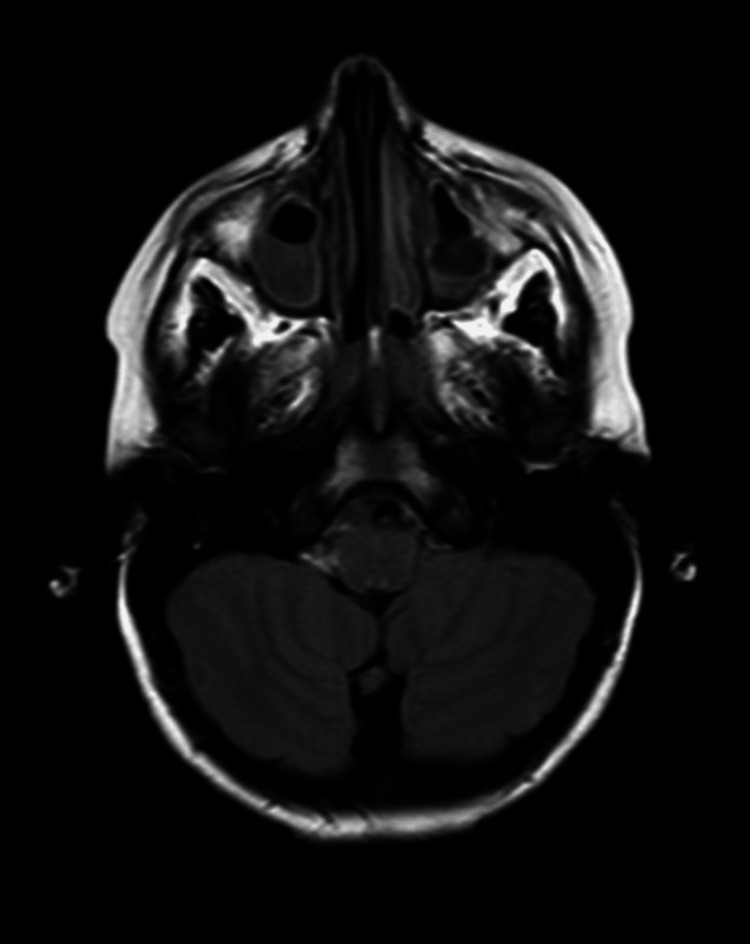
CT scan of the patient at the presentation CT scan image of the acute cerebellitis patient showed no abnormal structures, abnormal differentiation, fractures, or bleeding signs.
